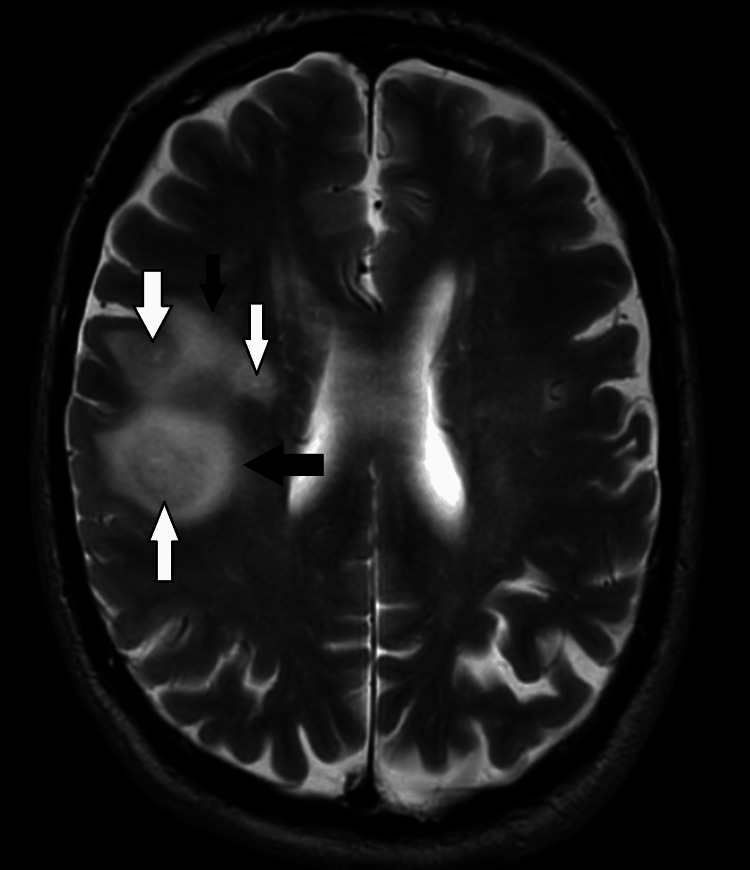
MRI of the head with contrast: T2-weighted sequence The white arrows show the necrotic core at the centre, while the black arrows show the perilesional edema - findings suggestive of GBM GBM: glioblastoma multiforme; MRI: magnetic resonance imaging
